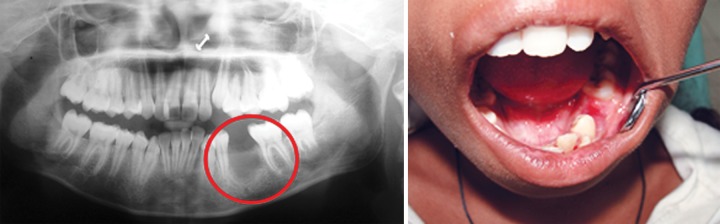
Postoperative radiograph and photographs
